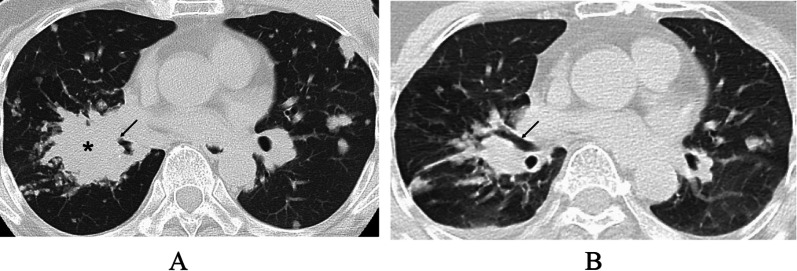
Computed tomography (CT) of the chest. A CT just before her first visit to our hospital showed multiple lung nodules. The largest lesion (asterisk) involved the right pulmonary hilum, causing stenosis of the bronchus intermedius (arrow). B Bevacizumab and paclitaxel therapy brought about marked regression of the lung metastatic foci and improvement of the bronchial stenosis (arrow)
